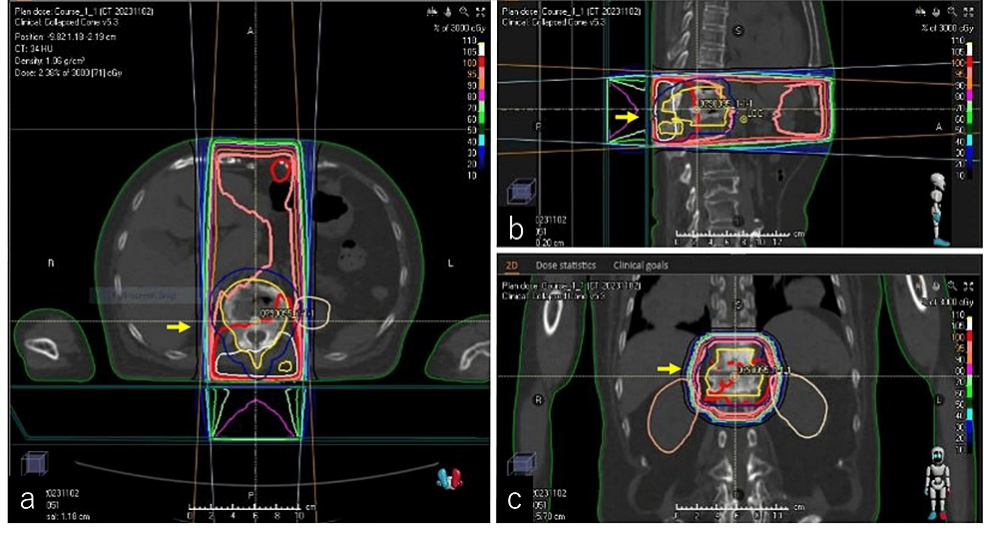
Irradiation field and dose distribution of radiation therapy. The RT plan was generated using the RayStation treatment planning system (RaySearch Laboratories AB, Stockholm, Sweden). The radiation therapy fields are displayed in axial (a, yellow arrow), sagittal (b, yellow arrow), and coronal (c, yellow arrow) images. Patient consent for treatment was obtained. We administered RT with a total dose of 30 Gy in 10 fractions over a period of two weeks. RT was delivered using 6- and 10-megavoltage equipment through a multileaf collimator by three-dimensional conformal RT from two anterior and posterior quadrants. Gross tumor volume was defined as the primary tumor in the 11th and 12th thoracic vertebrae based on the RT-planned CT. The CTV was defined as the 11th and 12th thoracic vertebrae. The planning target volume encompassed the CTV plus 1.0-cm margins, extending to include the upper part of the 1st lumbar vertebra.
